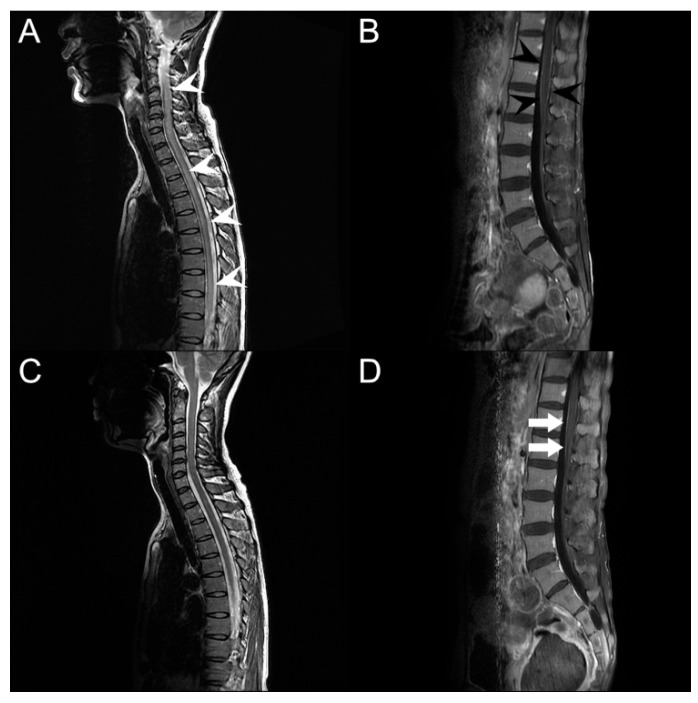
Spine magnetic resonance imaging. (A) T2-weighted sagittal imaging on admission shows abnormal intramedullary hyperintensity lesions from C1/2 to conus medullaris (white arrowheads). (B) T1-weighted sagittal gadolinium-enhanced imaging shows enhancement of pia mater on the conus medullaris (black arrowheads). (C) Intramedullary hyperintensity is improved after treatment. (D) Enhancement of pia mater remains even after treatment (white arrows).
